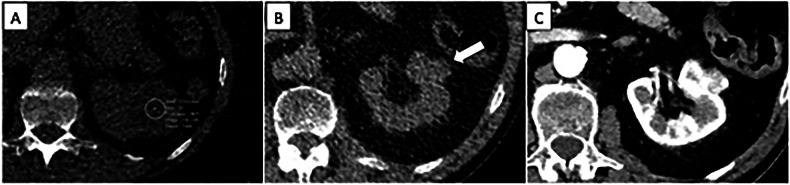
Challenges associated with the characterization of renal lesions. A Hypoattenuating lesion of the left kidney whose cystic or solid nature cannot be assessed due to a large deviation in attenuation measurement. B Exophytic lesion of the left kidney with high attenuation (white arrow); the extrathoracic IF was reported and evaluated by contrast-enhanced CT (C), which confirmed a lesion representing a stage I clear cell renal cell carcinoma. The detection of such a small exophytic renal lesion was due to a very attentive image review: in the setting of LCS, it can be debated if it should be considered a missed diagnosis if unmentioned
